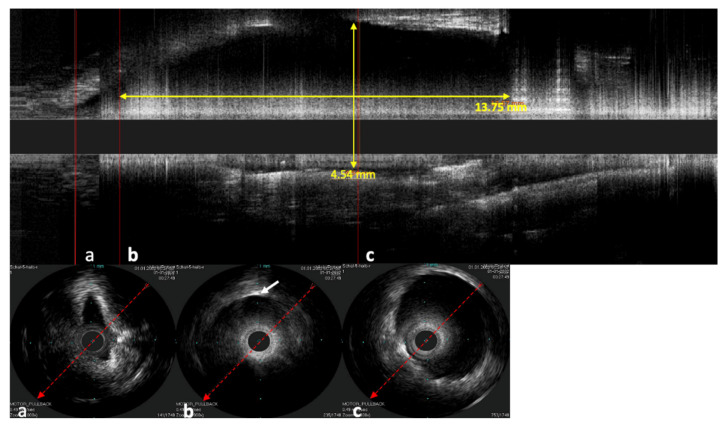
Sonographic image of the stented ET, performed with the Refinity ST probe. The upper image shows the longitudinal view of the scan. Parts (a–c) show the respective cross-sections of the scans. Part (a) shows the area in front of the stent, where the tubal lumen widens toward the stent. Part (b) shows the initial area of the stent where the first stent strut can be seen sonographically (indicated by white arrow). Part (c) shows the cross-section in the middle section of the stent. The cross-section here was measured to be 4.54 mm. In the longitudinal view, a length of 13.57 mm was measured.
